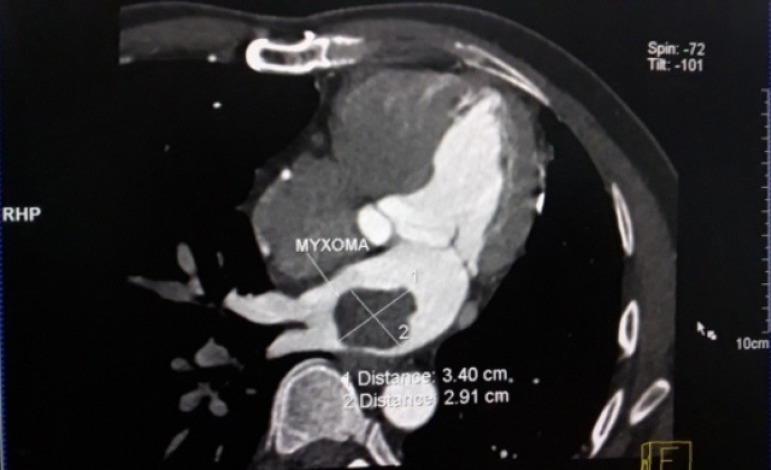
Computed tomography scan showing large left atrial intracardiac mass.
